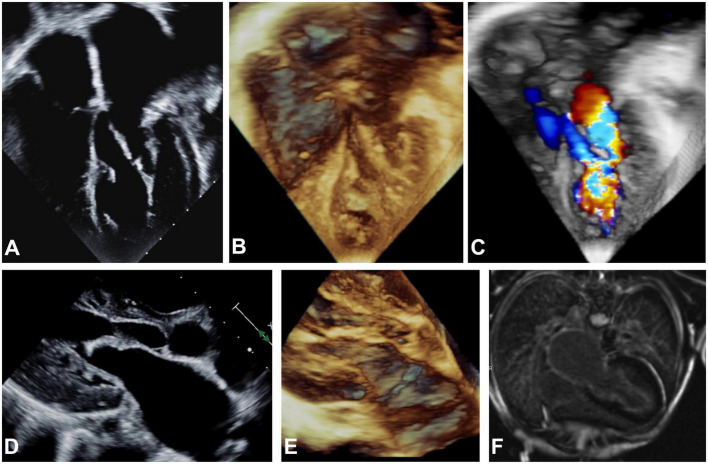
Echocardiographic apical 4-chamber view (A–C), parasternal long axis (D,E) and MRI (F) imaging before EFE resection. 2D (A,D) and 3D (B,C,E) echocardiography showing hyperechogenic thickening of the endocardium on atrial, mitral valve, LV and LVOT level consistent with generalized EFE in the left sided structures of the heart is shown. Severe dilation of the left atrium can be noted. (C) Severe turbulences are detected by color Doppler in all left sided structures affected by EFE as compared to physiological color Doppler appearance in the right sided structures of the heart that are not affected by EFE. (F) Late gadolinium enhancement on endocardial level encapsulating all structures of the left atrium and ventricle is depicted.
